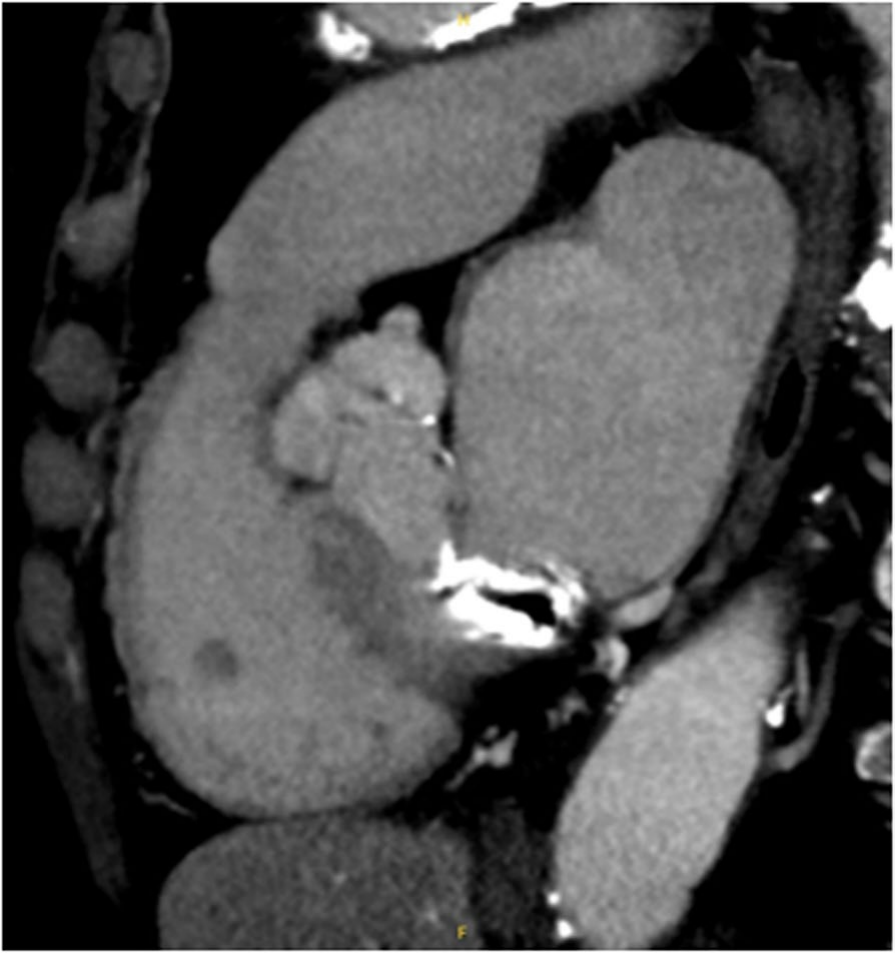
Preoperative computed tomography shows MAC in the mitral valve. MAC: mitral annular calcification
